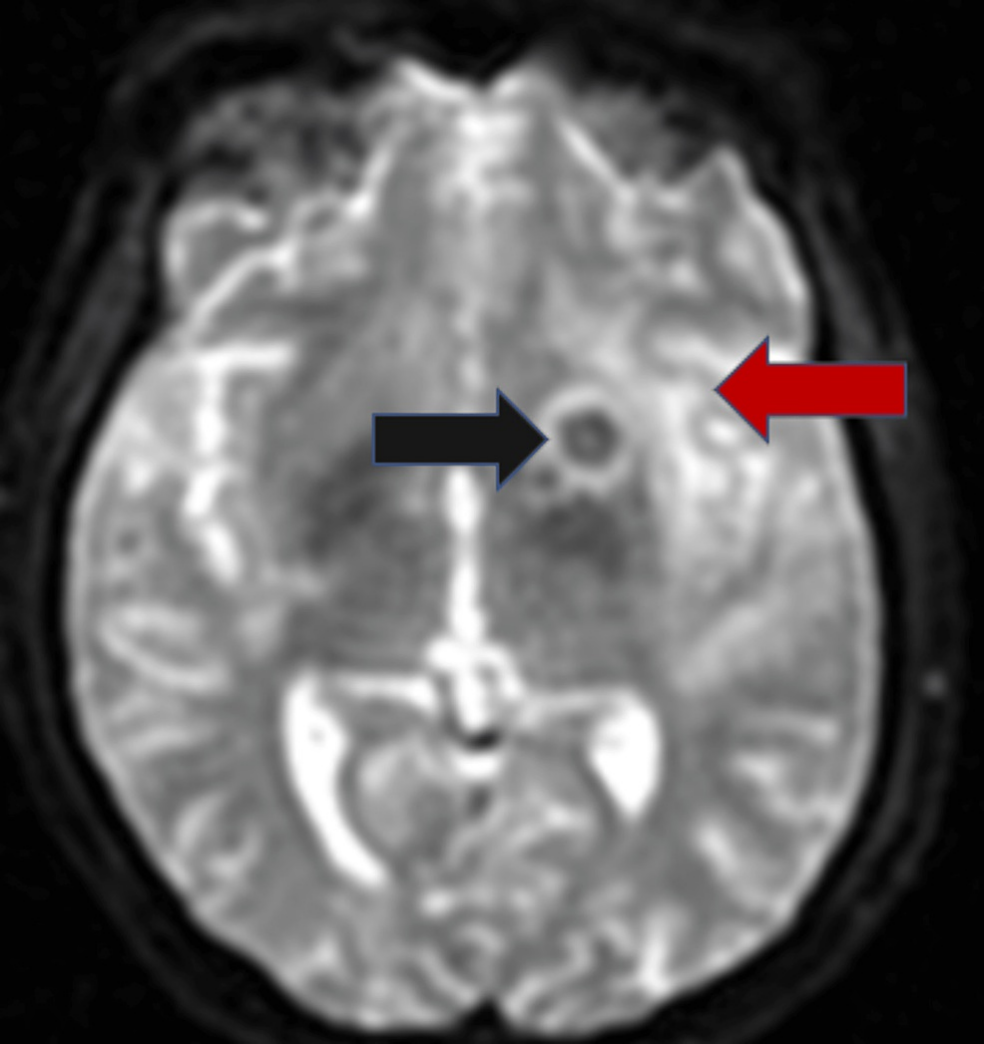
Diffusion-weighted imaging of T2-weighted MRI of the brain in axial projection The black arrow represents tuberculoma showing no foci of restriction on diffusion-weighted imaging. The red arrow represents a hyperintense area in the left perisylvian cortex showing foci of diffusion restriction, likely post-ictal edema.
